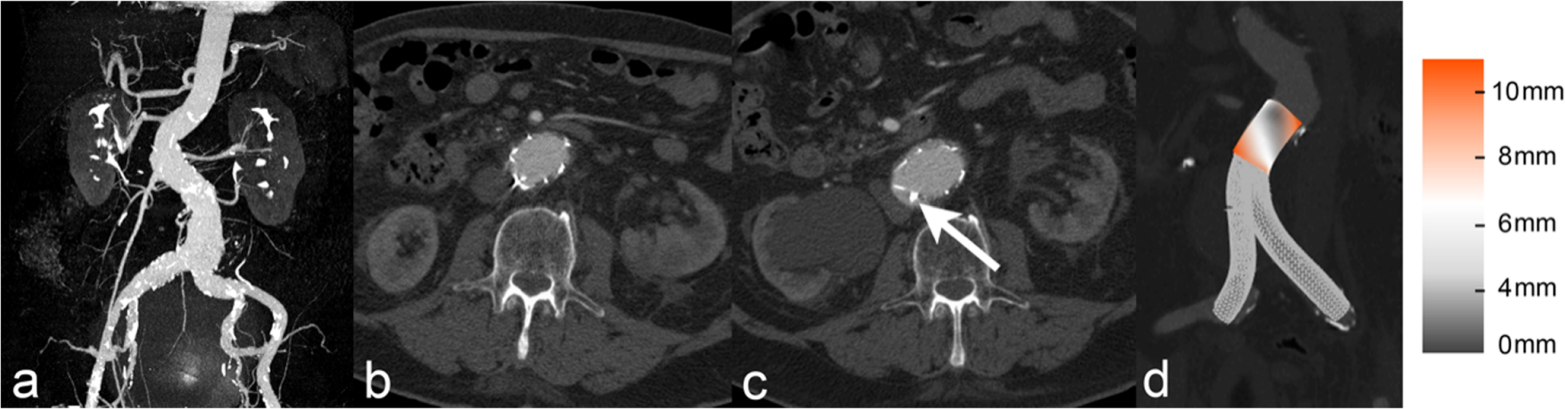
a–d 83-year-old male patient with evident angulation and wide diameter of the infrarenal neck on pre-procedural CTA-maximum intensity-projection images (a). In comparison to the pre-discharge CTA (b), there is an obvious lateralization of the superior mesenteric artery and the abdominal aorta with consecutive compression of the inferior vena cava after 4 years of follow-up (c). Further, the aortic neck diameter increased from 29 to 36 mm with consecutive sealing loss at the dorsal circumference of the proximal anchoring zone and occurrence of a type 1a endoleak (arrow). Significant stent-graft surface movement (> 9 mm) at the proximal anchoring zone is visualized on 3D matched surface models of postprocedural and final follow-up CT (d)
